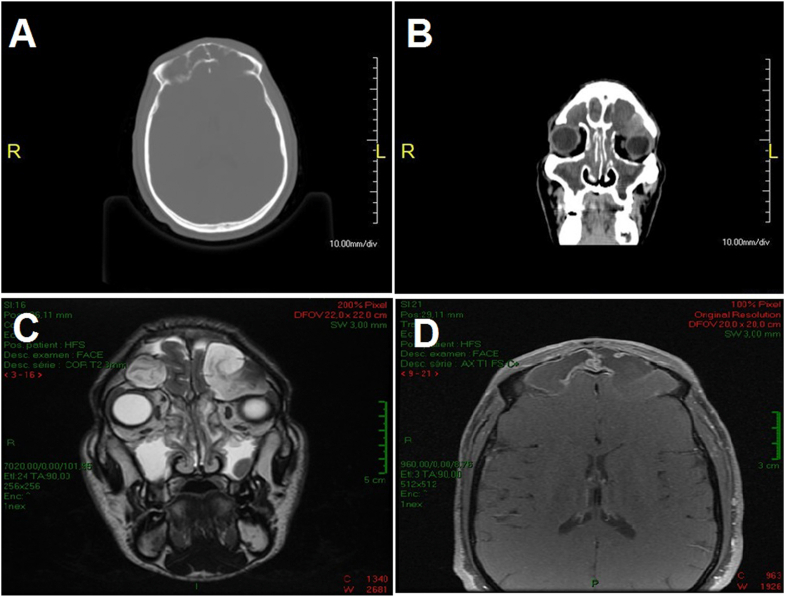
(a) CT bone window in axial section showing total filling of the frontal sinuses with blown appearance of the sinus walls, (b) CT parenchymal window in coronal slice showing 2 frontal mucoceles with left orbital extension, (c) Coronal T2 weighted MRI showing an expansive process of the frontal sinuses with hyperintensity lowering the roof of the left orbit, and (d) Axial T1-weighted MRI after injection showing a hypointense lesion of the frontal sinuses, enhancing peripherally with bilateral endocranial extension.
